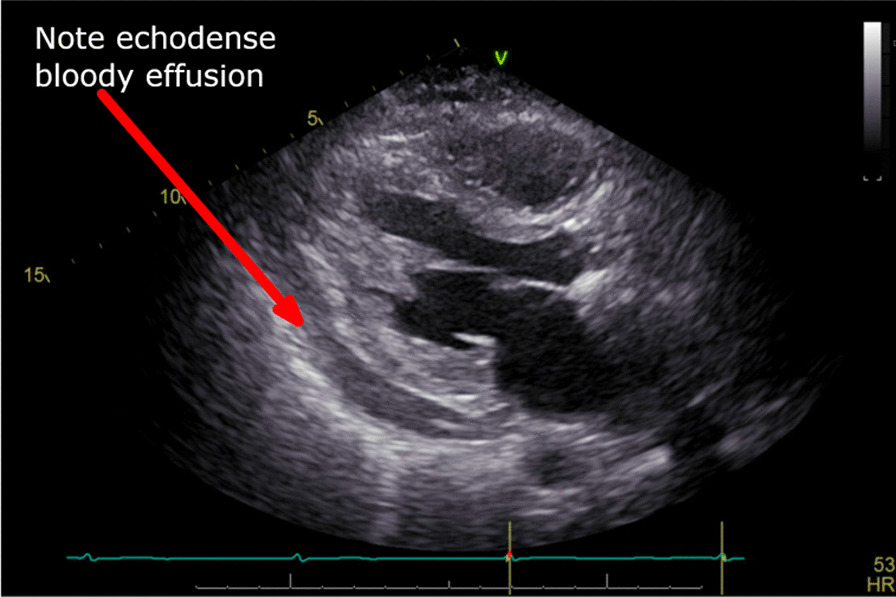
Patient 3 transesophageal echocardiogram showing echo-dense pericardial effusion suggestive of bloody effusion and tamponade
